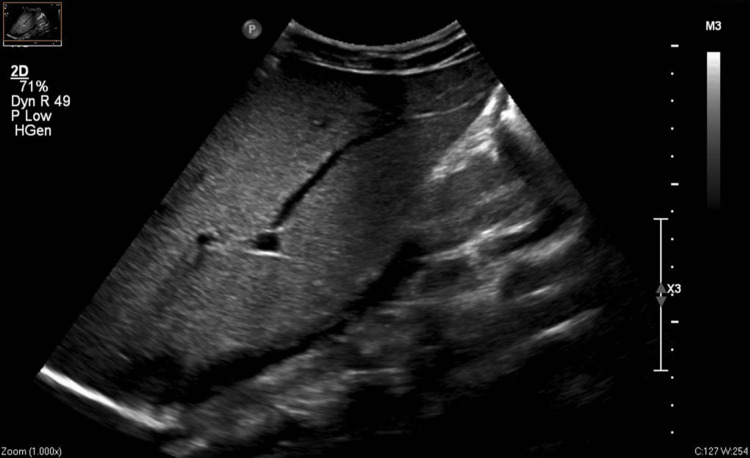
Finding of hepatomegaly on ultrasonography
